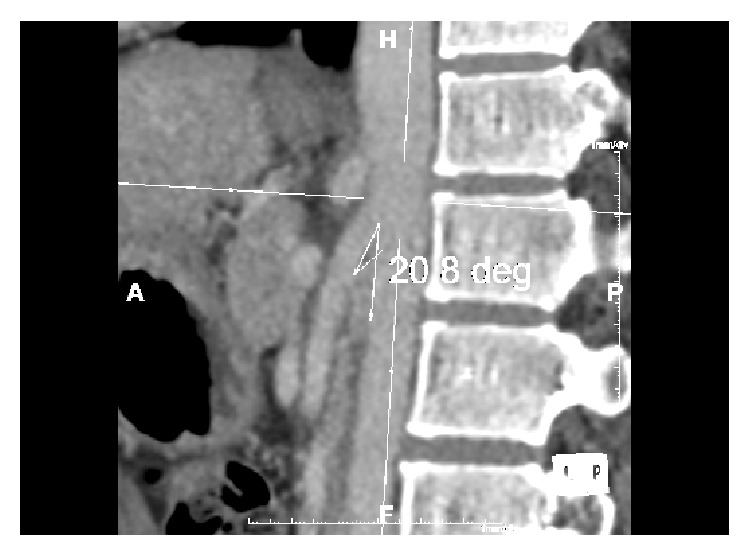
Sagittal contrast CT of our patient showing a mesoaortic angle of 20.8 degrees.
